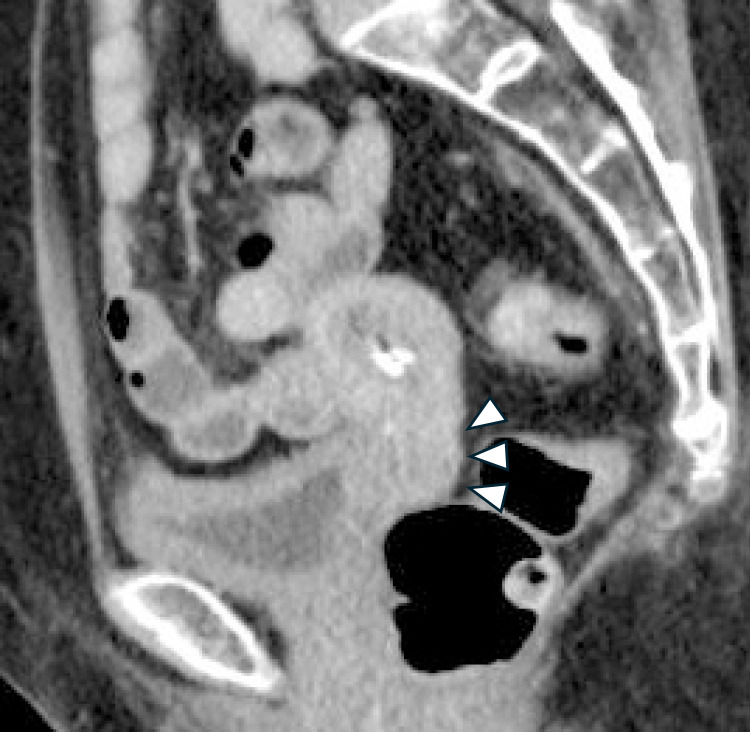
Non-contrast sagittal CT of the pelvic region showing a reduced cervical mass after cemiplimab treatment (arrowhead).
